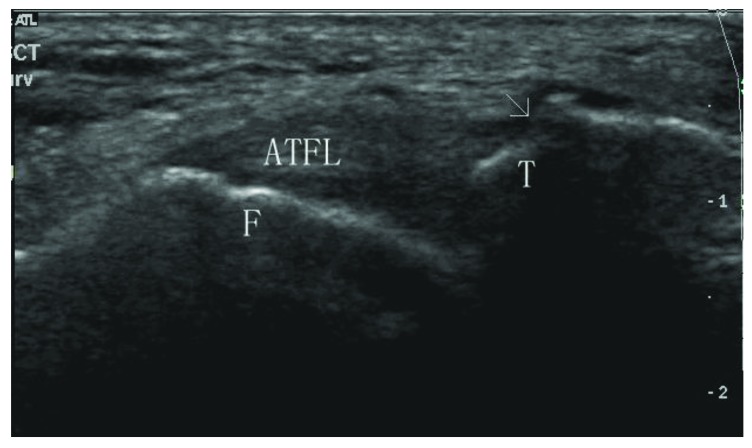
Sonogram shows a total tear at the talar side (type IIIB). ATFL: anterior talofibular ligament; F: fibula; T: talus; Arrow: ligament tear at the talar side.
